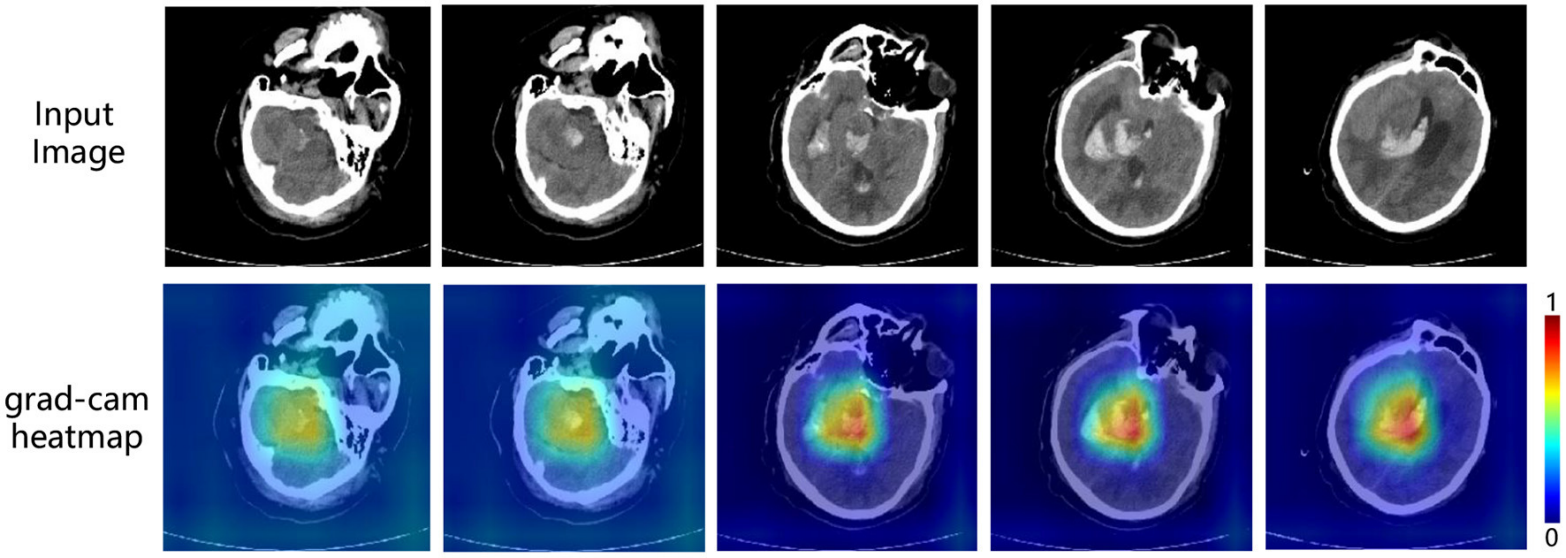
Interpretable visualization of our multi-task framework. The images are five slices taken from a 3D CT scans at medium intervals (*t* = 5).
